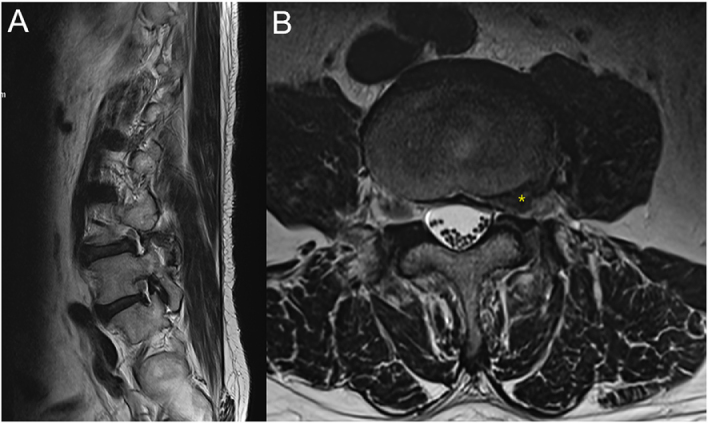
The preoperative magnetic resonance image (MRI) of the lumbar spine. (a) Sagittal; (b) axial cut on T2WI. The MRI showed disc extrusion (a yellow asterisk) in the left extra-foraminal zone with cranial migration, causing L4 nerve root compression.
